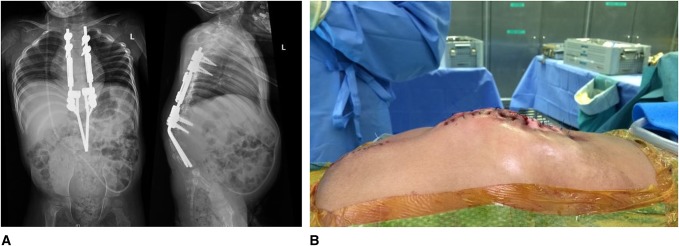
A, Immediate postoperative AP and lateral radiographs showing the correction of the kyphosis. Traditional growing rod construct is noted in the thoracic spine, connected by domino correctors to the distal construct. The convergence of the distal rods helps resist implant pullout. B, Intraoperative image immediately after wound closure. The skin closed easily without flaps or preoperative skin expanders.
